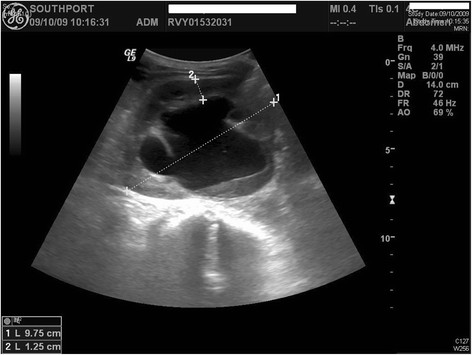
Ultrasound scan of urinary tract repeated ten days later after the patient had received antibiotic: there was less debris within the hydronephrotic right kidney than at the previous scan. There was however still significant hydronephrosis with antero-posterior diameter of right renal pelvis being 5.7 cm.
